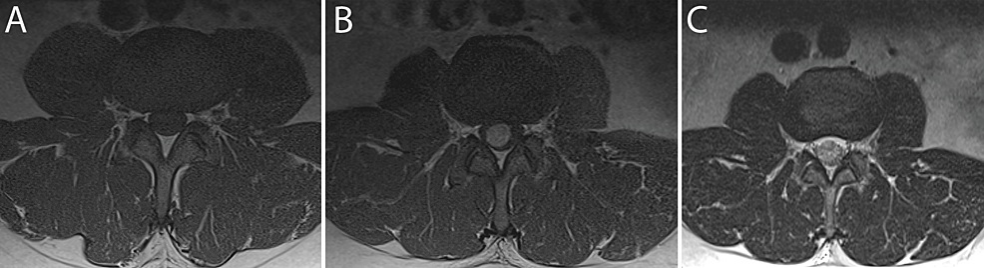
Preoperative MRI of the cauda equina showing: (A) axial T1 without contrast; (B) axial T1 with contrast; (C) axial T2.
